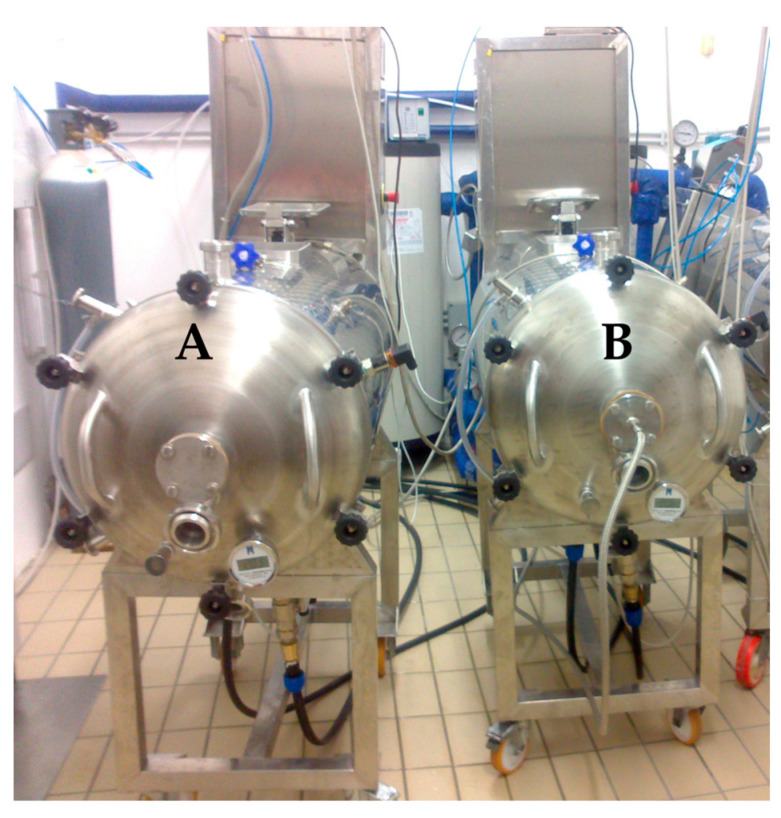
Rotary wine fermenter pilot plants: (A) control; (B) with ultrasound system.
